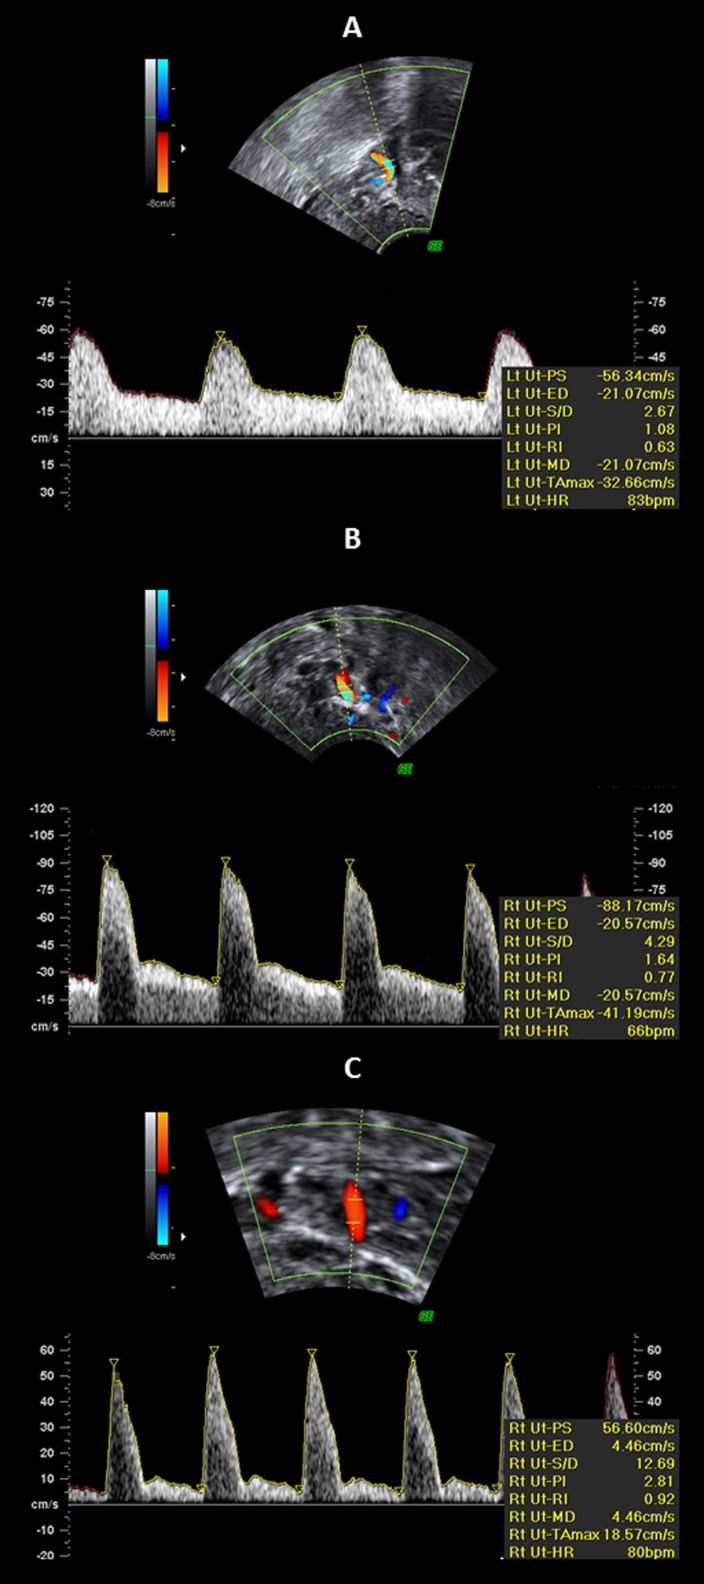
Doppler shift spectra recorded from the uterine arteries at 1 (A), 4 (B) and 8 (C) weeks postpartum. (A) Waveforms with velocities gradually decreasing from the systolic peak and with continuous forward flow in diastole; (B + C) waveforms with a notch and with continuous forward flow in diastole. The pulsatility index (PI) is used as a measurement of impedance of the flow blood distal to the sampling point and is automatically calculated according to the formula
